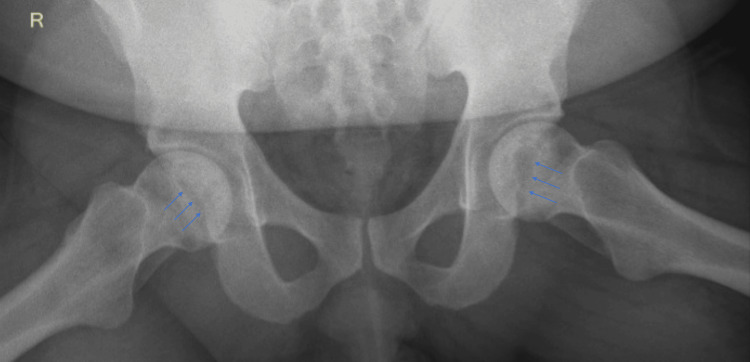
X-ray of the pelvis with serpiginous sclerosis with central lucencies at the femoral heads (blue arrows) suggesting osteonecrosis
